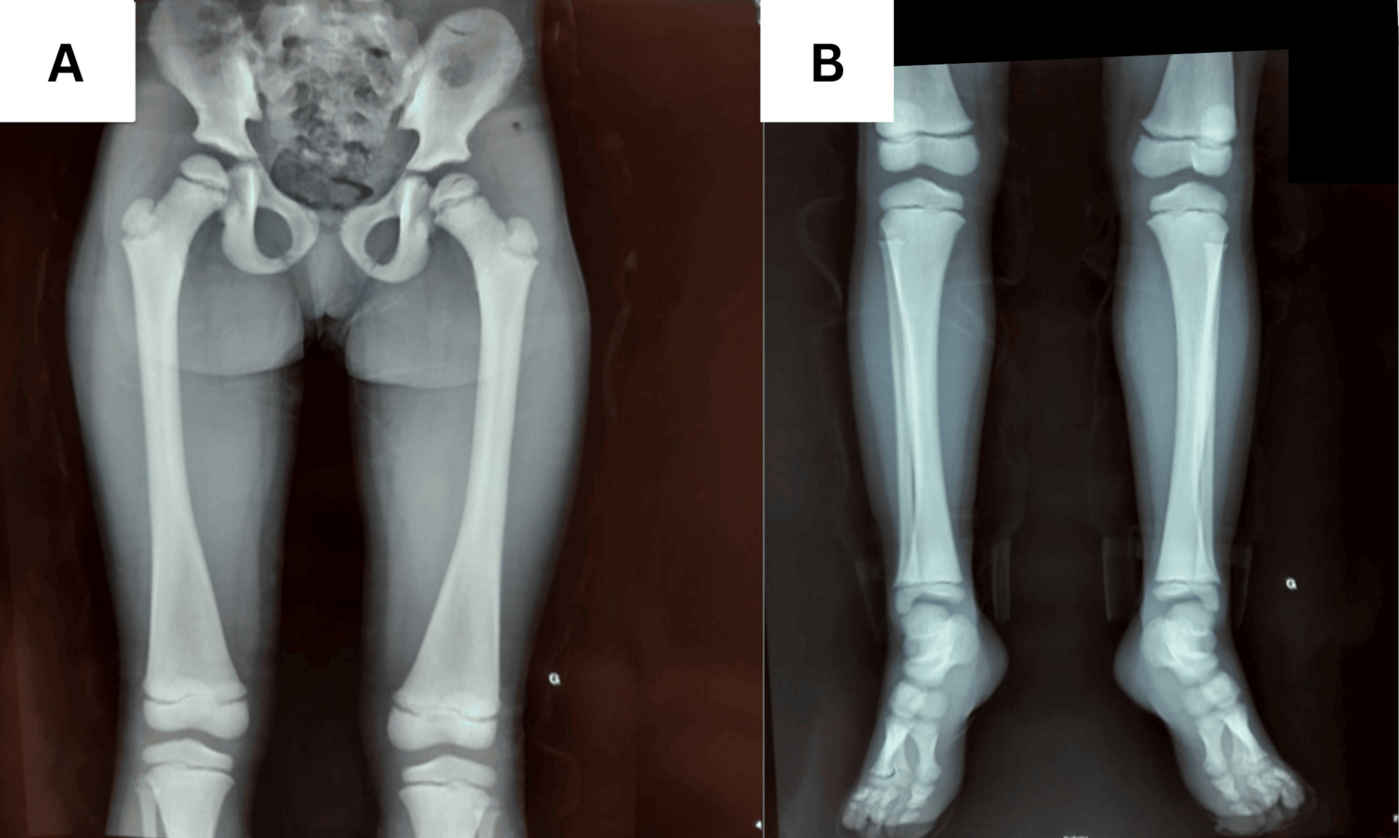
Radiograph of the pelvis and femurs (right and left) (A) and legs and feet (right and left) (B) in anteroposterior view showing increased bone density
